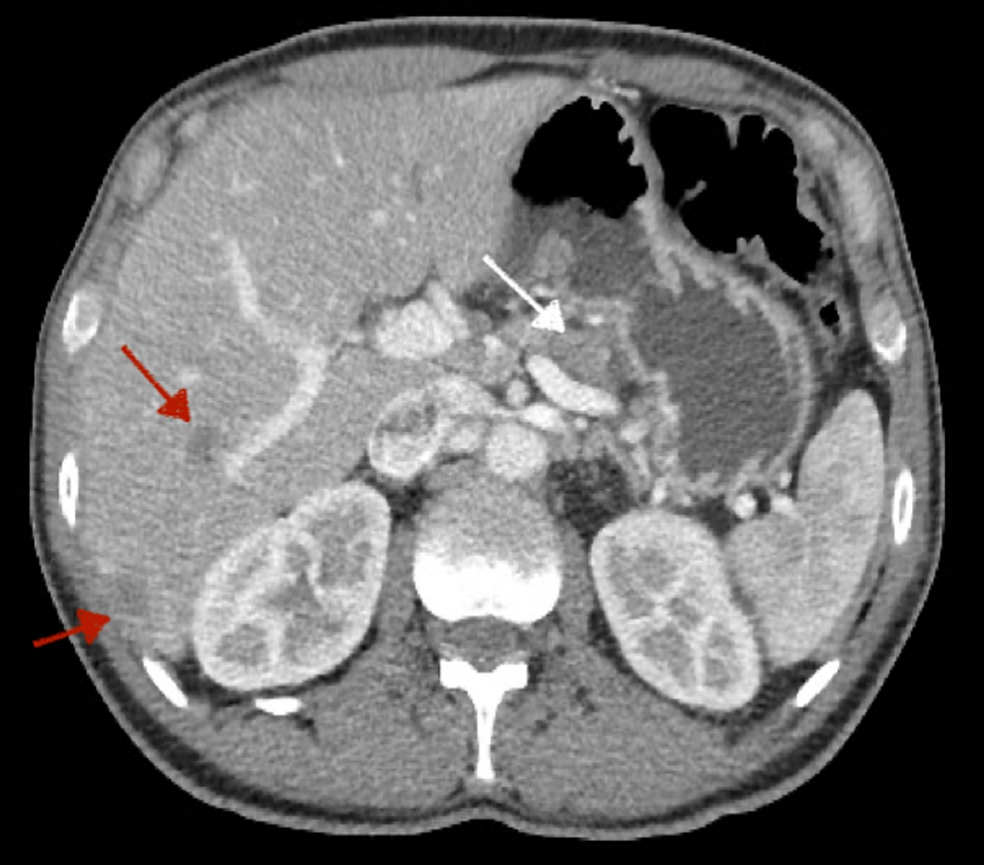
CT abdomen shows newly developed lesions in the liver (red arrows) after 12 months of starting second-line GNP (Case 3). The pancreatic mass showing interval regression (white arrow)
